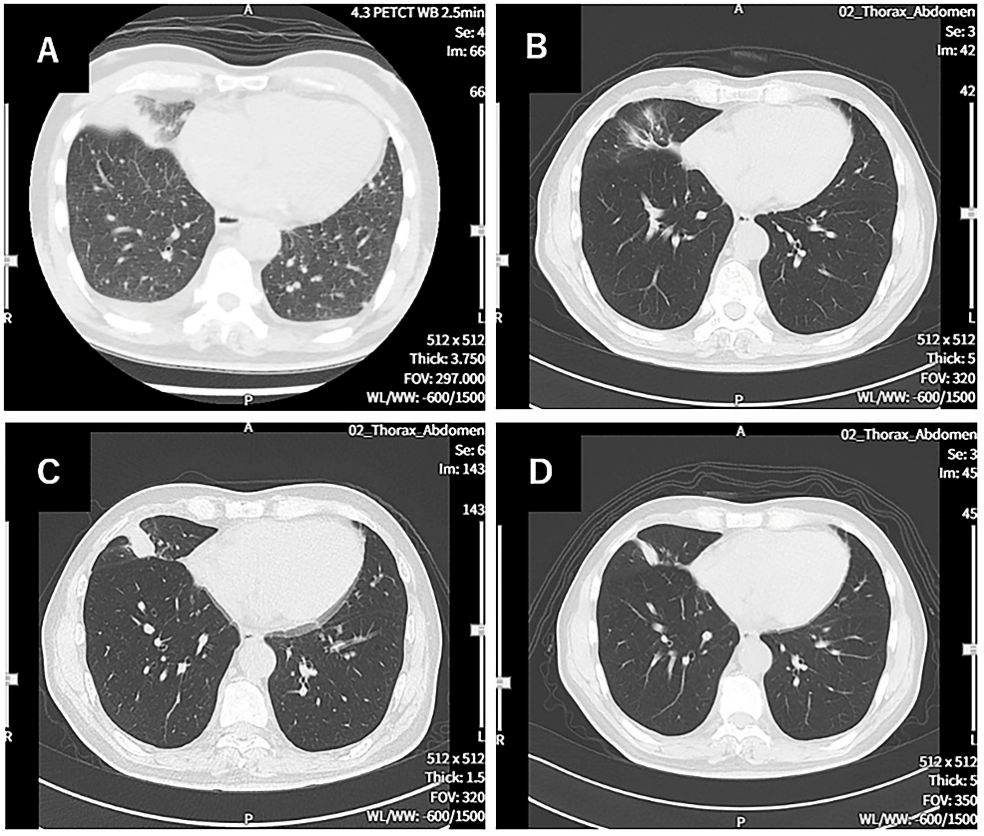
CT image of the chest A: CT image at diagnosis. Right and left pleural effusions were seen, along with the primary right lower lung cancer. B: CT image on the 114th day. The primary lesion has almost disappeared. C: CT image on the 213th day. The recurrence of the primary lesion was shown. D: CT image on the 338th day. The primary recurrent lesion has shrunken.
